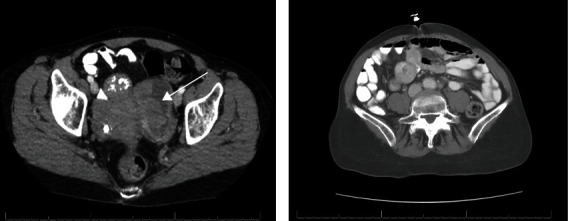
Comparative CT analysis of the patient's abdomen/pelvis before surgery, showing the ovarian mass (a, arrow) and in May 2023, where no abdominal/pelvic recurrence is identified.
